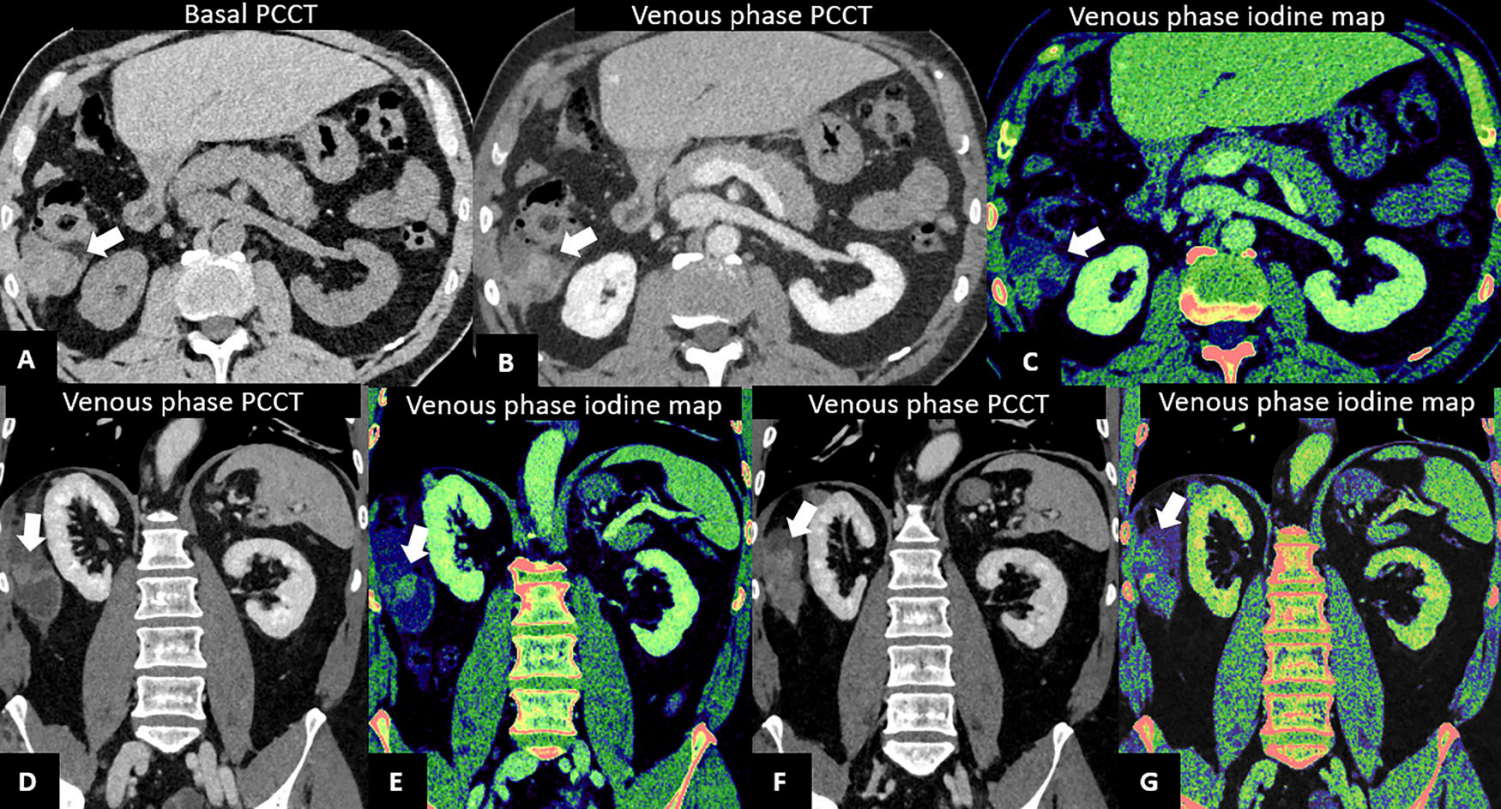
Peritoneum masses on PCCT. A 59-year-old man with a history of jejunal adenocarcinoma and liver metastasis, suspected of having a hematoma anterior to the right colon at the basal scan on EID-CT. Pre-contrast (A), post-contrast venous phase (B) axial and coronal views (D) show a fluid collection located in the right paracolic gutter, with a 3 cm solid tissue (white arrows in A–G), hyperdense on both basal and post-contrast scan, with evidence of iodine uptake on iodine maps (C, E), resulting referable to disease relapse. PCCT performed after 3 months demonstrated a significant increase in size and iodine uptake of the pathological tissue in the venous phase (F), more clearly visible in the iodine map (G)
